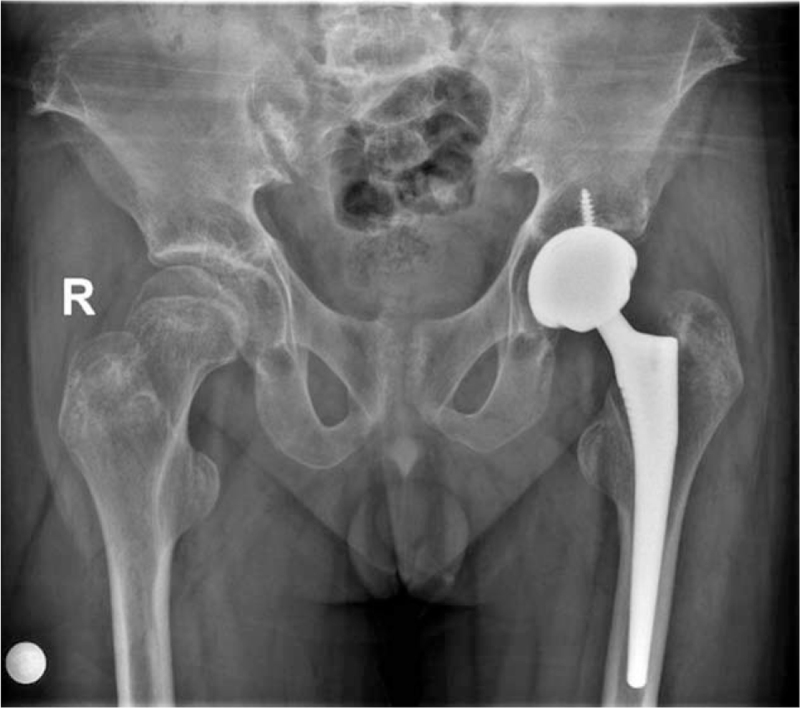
Anteroposterior pelvic radiograph was taken after total hip arthroplasty.
